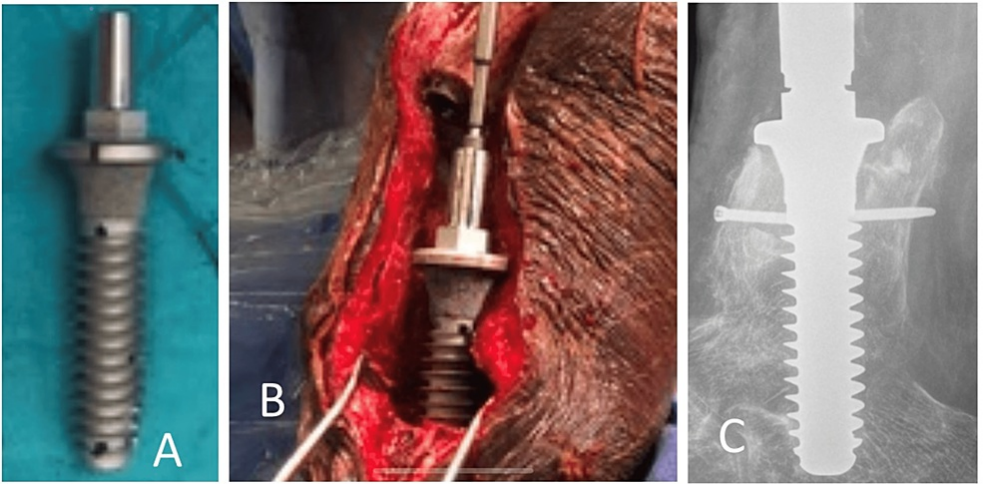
Intraoperative photographs (A, B) and postoperative radiograph (C) of the custom stem based on a senior author's design.
